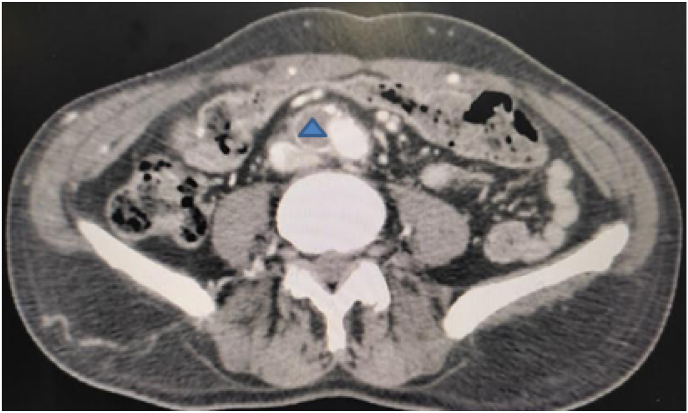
CT Angiography in axial view showing a massive thrombus in right common iliac artery (triangle).
